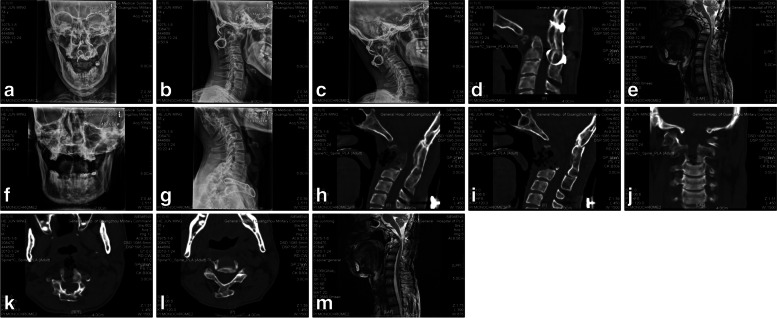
Shows the examination results of a 35-year-old male with the malunion after the thrice operations of atlantoaxial dislocation. He was treated with posterior occipitoatlantoaxial cable removal followed by second-stage transoral C2 corpectomy. X-rays (see a, b and c) show space occupying of steer wire internal fixation and good posterior plate fusion in occipital and atlantoaxial segments, without any instability or displacement. The CT results (see d) reveal firm posterior plate fusion in occipital and atlantoaxial segments, with Brooks steer wires fixed inside. Moreover, the sagittal diameter of the spinal canal is markedly narrow. MRI results (see e) show significant compression and partial degeneration of spinal cord. As shown in f and g, the inside steer wires of occipital and atlantoaxial segments have been removed completely. Besides, the posterior bone plate is of structural integrity without obvious fracture or dislocation. The CT results (see h, i, j, k and l) show the odontoid process of C2 vertebra has been removed, with only a small amount of bone residue. MRI results (see m) show that the compression of spinal cord at atlantoaxial level is significantly less than that before operation. The sagittal diameter of spinal canal is effectively restored. Moreover, the flow of cerebrospinal fluid in the posterior dural sac is obviously unobstructed, and there is no significant change in the partial degeneration of the spinal cord
